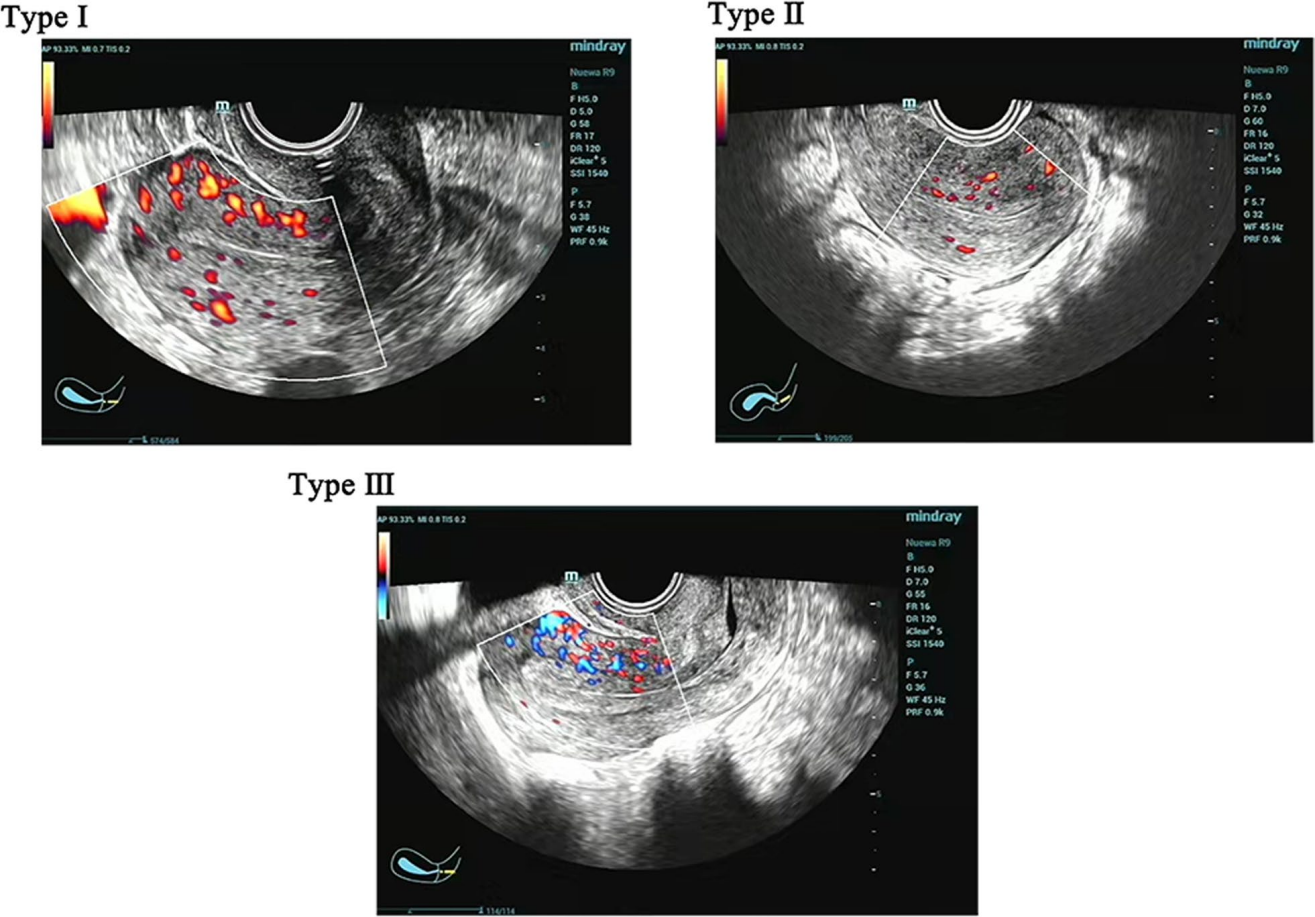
The color ultrasonography image of the type of endometrial blood flow
